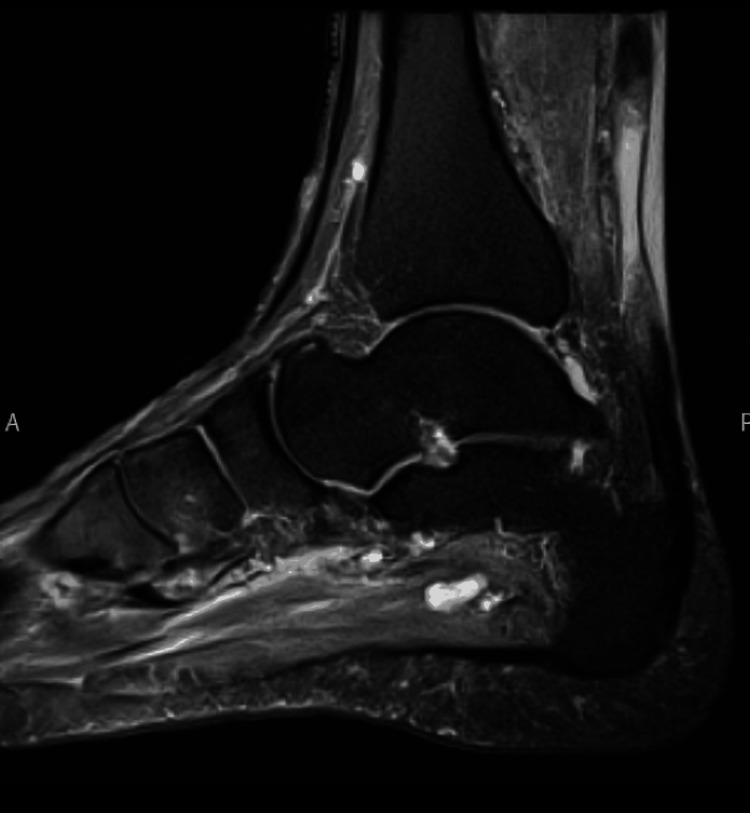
Achilles tendon (right) sagittal PD MRI image PD: proton density; MRI: magnetic resonance imaging
